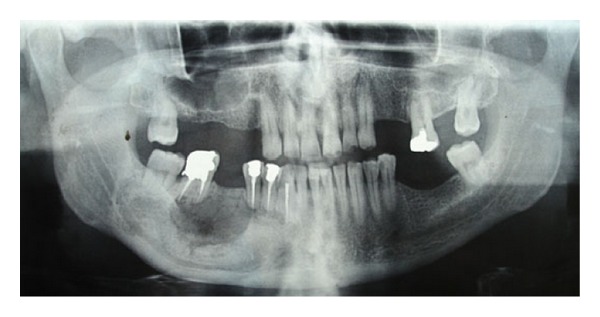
Panoramic radiograph; 18 months after operation.
